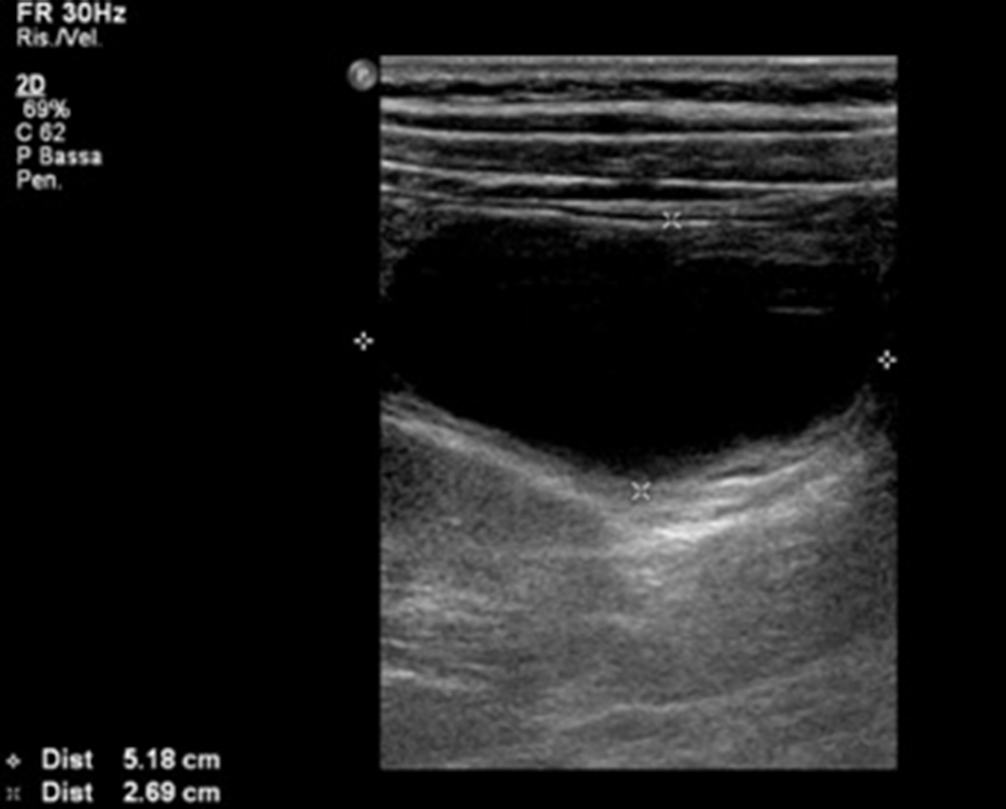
The eco-FAST shows formation of 51 x 27 mm with mainly anechoic content, equipped with a triple-layer wall, movable on the deep planes. FAST, Focus Assessment Sonography for Trauma.
